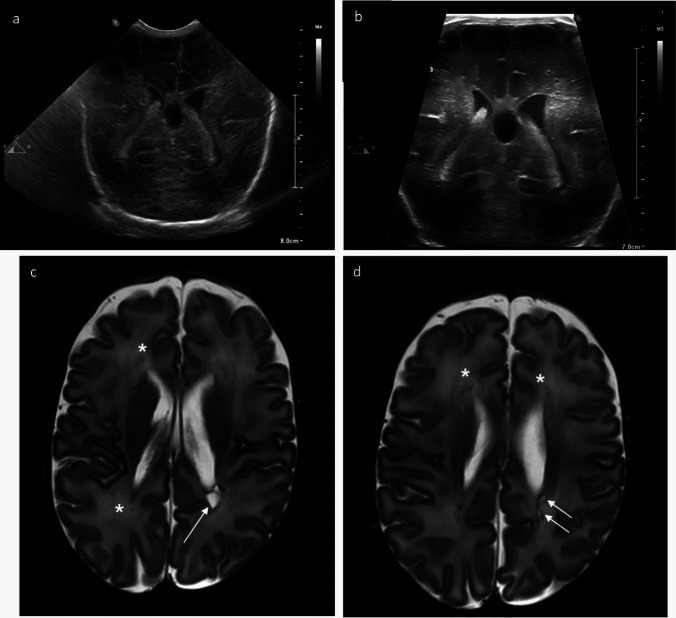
Microcystic periventricular leukomalacia (PVL) and ex vacuo dilatation of lateral ventricles in a preterm infant (29 weeks gestation) with in utero twin-to-twin transfusion. a, b Posterior coronal scans, 15 days of life. Hyperechoic changes affecting bilateral periventricular white matter that appear diffuse and homogeneous when cranial ultrasound is performed with the micro-convex transducer (a). By contrast, using the very high-frequency linear transducer hyperechoic lesions appears more inhomogeneous, and multiple small cysts are visible in the left periventricular white matter (b). MRI T2-weighted sequence (c, d) detected focal punctiform hypointensities in the white matter adjacent to the roof of both middle cells (asterisks). Cavitated lesions in the white matter adjacent to the posterior profile of the left lateral ventricle (arrows). Squared appearance of the posterior aspect of both ventricles and loss of the tapetum, indicative of white matter injury
